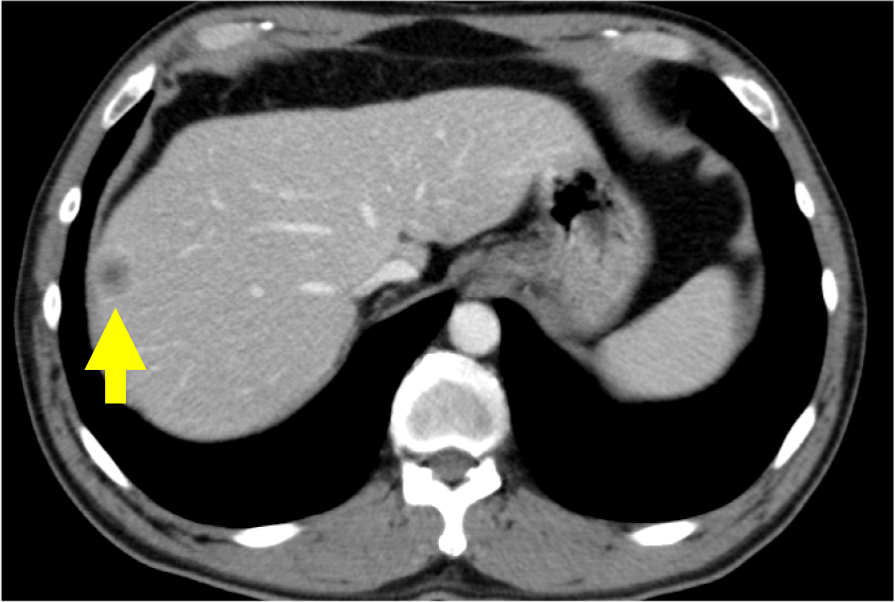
Contrast-enhanced CT. Contrast-enhanced CT showed a ring-enhanced tumor in the right hepatic lobe (arrow)
